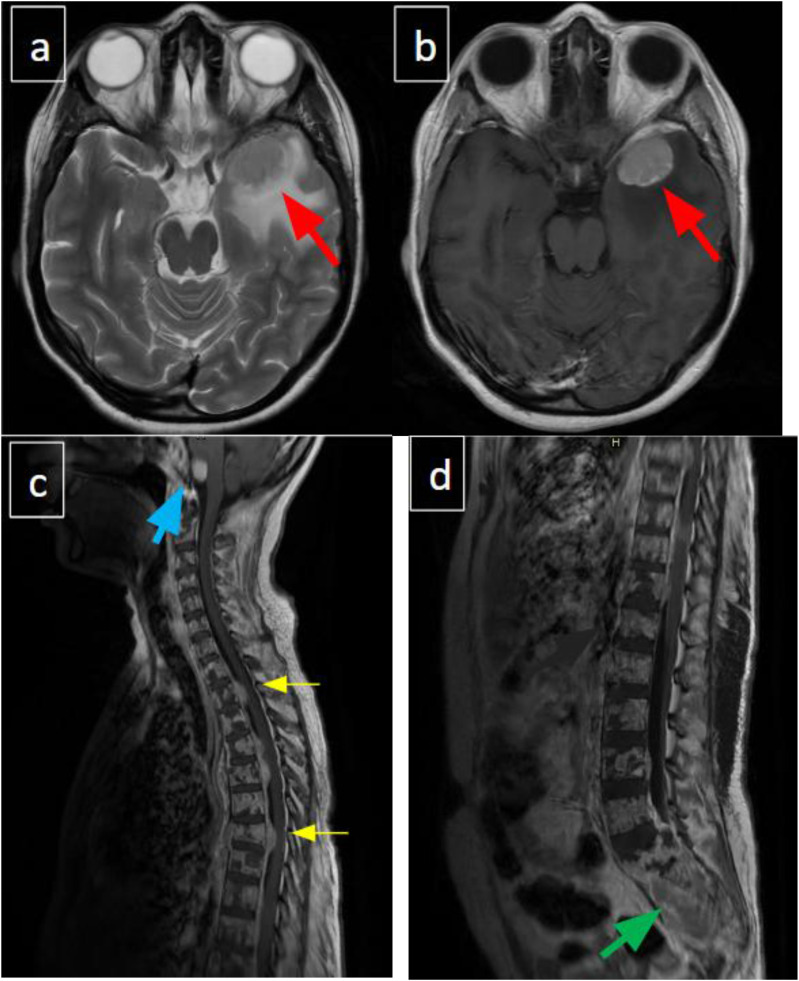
(A-D). Contrast-enhanced MRI. (A and B) Dural-based enhancing metastasis in the left middle cranial fossa with temporal edema. (C and D) Diffuse vertebral metastatic infiltration and large sacral lesion
